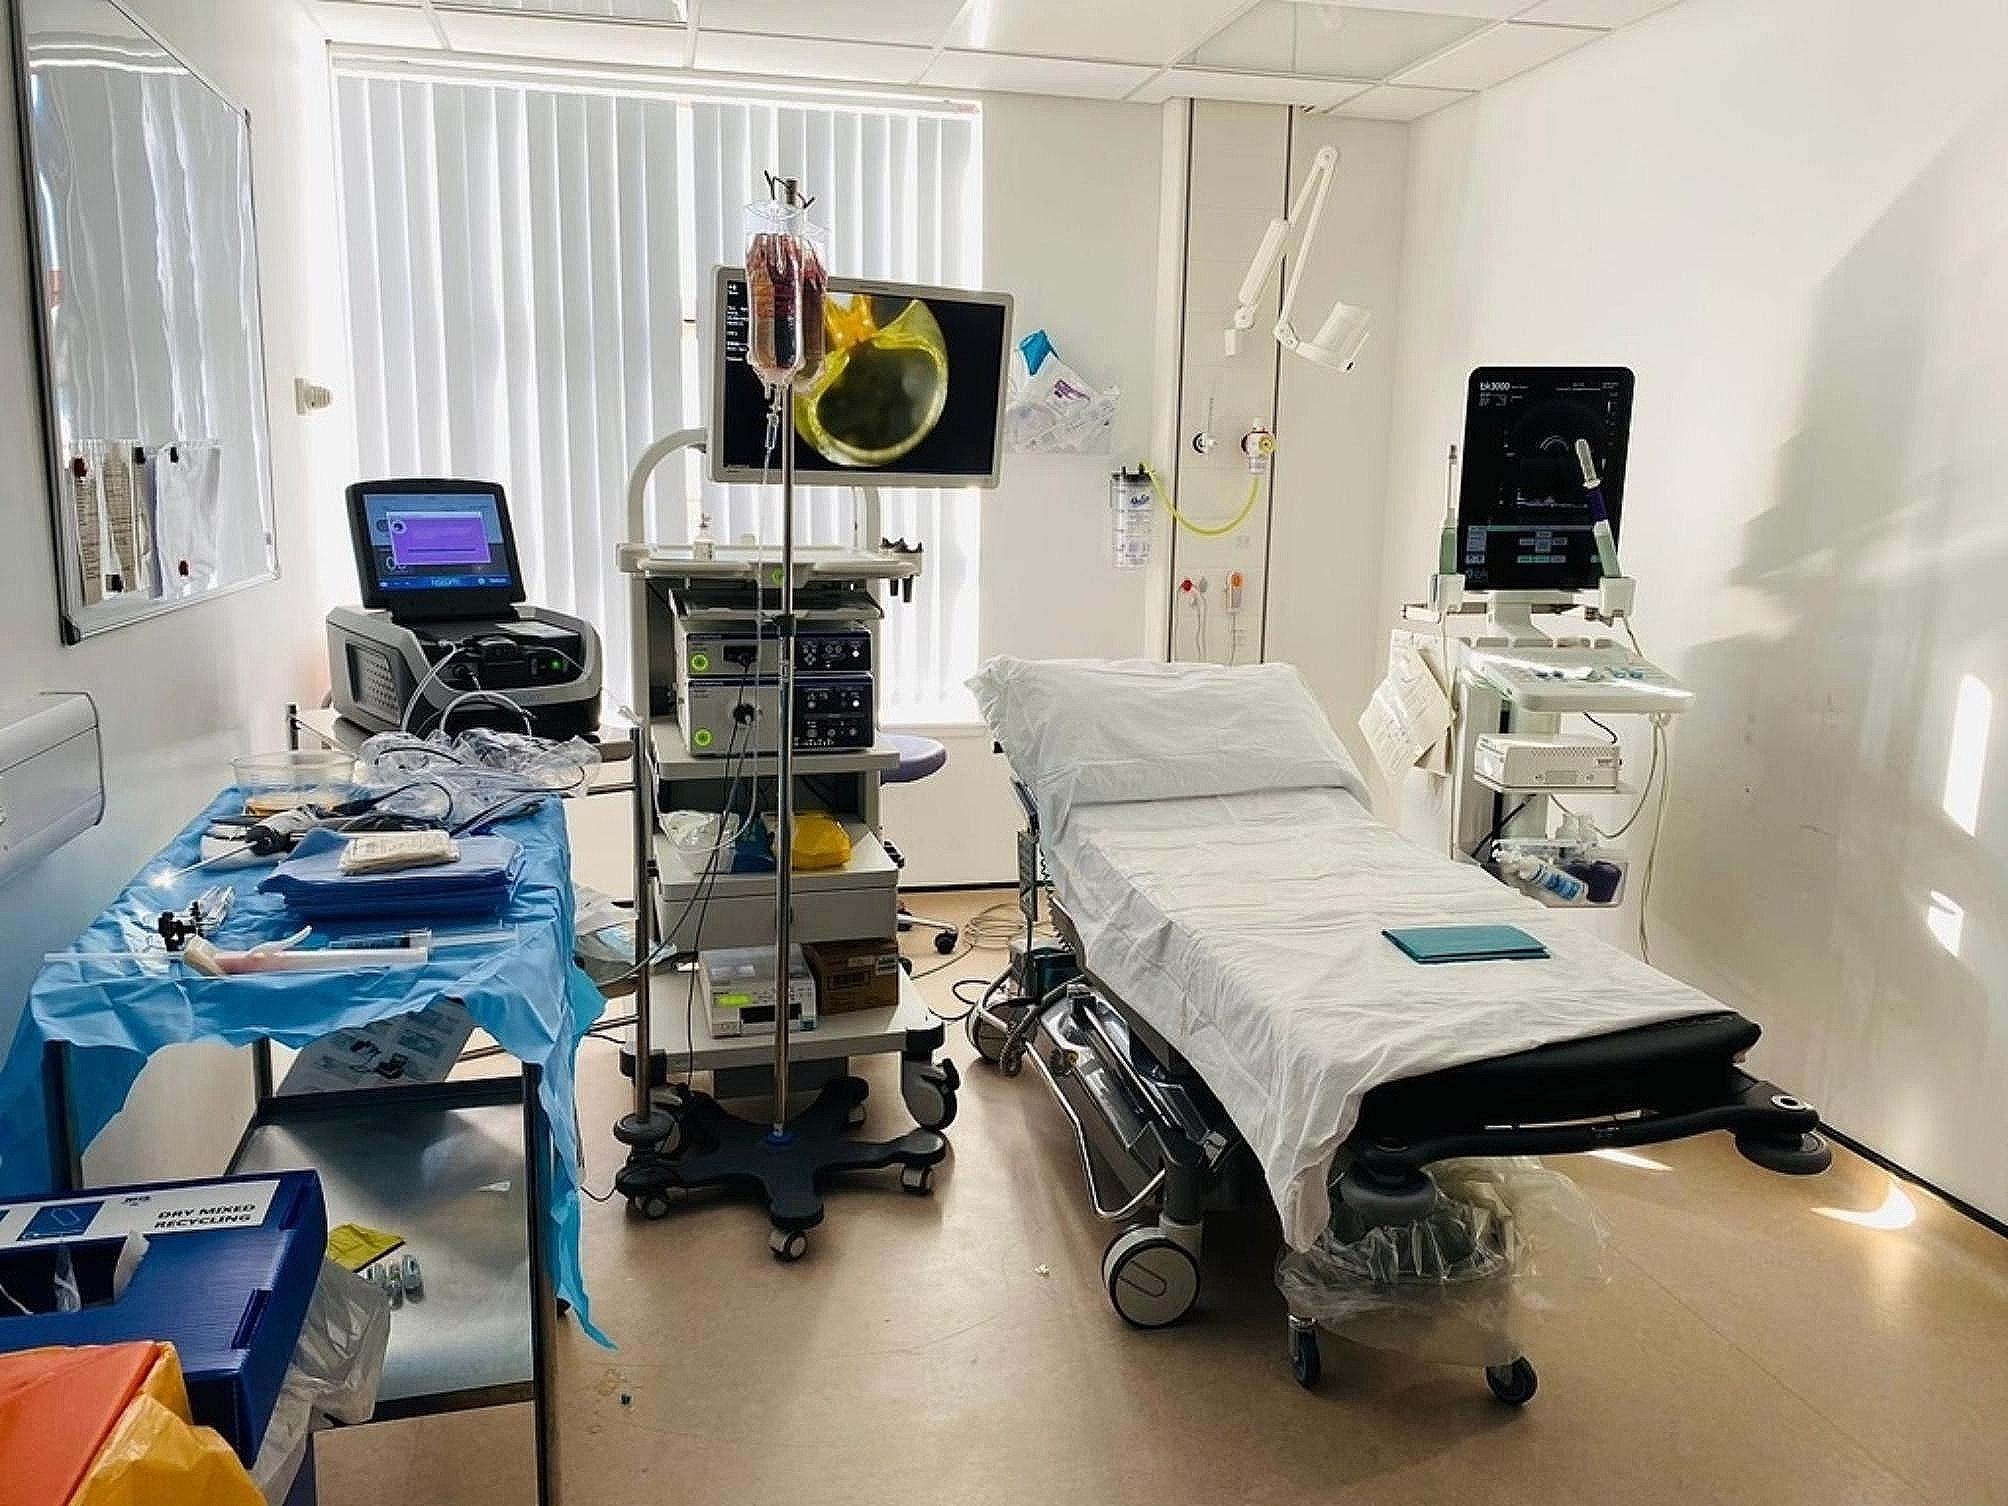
Room setup with ultrasound machine to the right of the couch, and cystoscopy stack, IV drip stand, Rezūm™ machine and scrub trolley to the left
